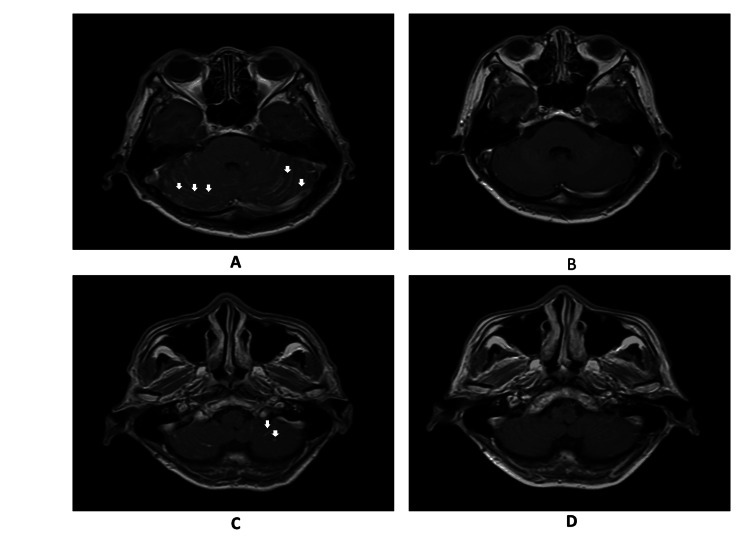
Axial brain MRI images before and after induction therapy A: Pre-induction axial T1-weighted image at the level of the posterior fossa demonstrating nodular leptomeningeal enhancement in bilateral cerebral hemispheres. B: Corresponding post-induction axial T1-weighted image showing markedly reduced leptomeningeal enhancement. C: Pre-induction axial T1-weighted image at a lower level demonstrating enhancing nodules at the base of the left cerebellar hemisphere. D: Post-induction axial T1-weighted image showing partial resolution following induction therapy.
